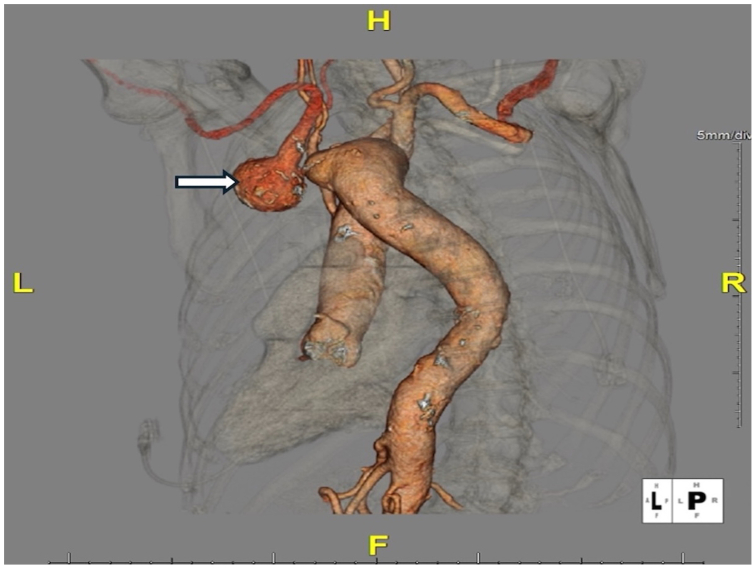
Preoperative three-dimensional reconstruction of chest computed tomography angiography (CTA) showing a right-sided aortic arch with an aberrant left subclavian artery arising from a Kommerell diverticulum (*white arrow*). The saccular aneurysmal dilation at the origin of the left subclavian artery is visualized posterior to the esophagus.
